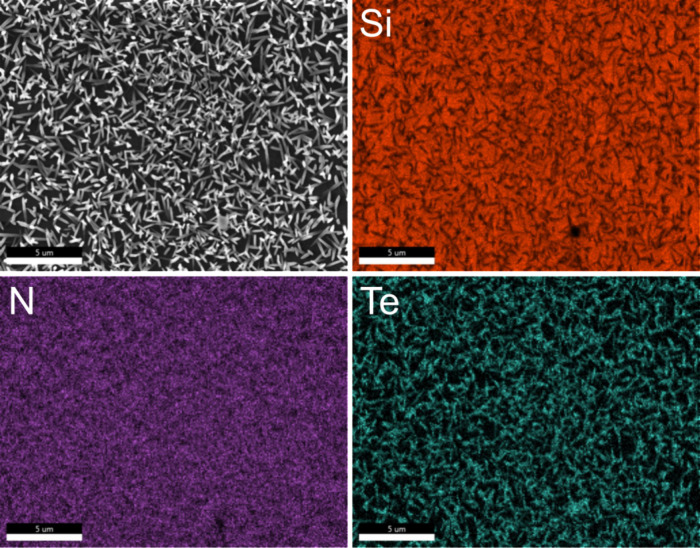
Energy-dispersive X-ray mapping of Te
